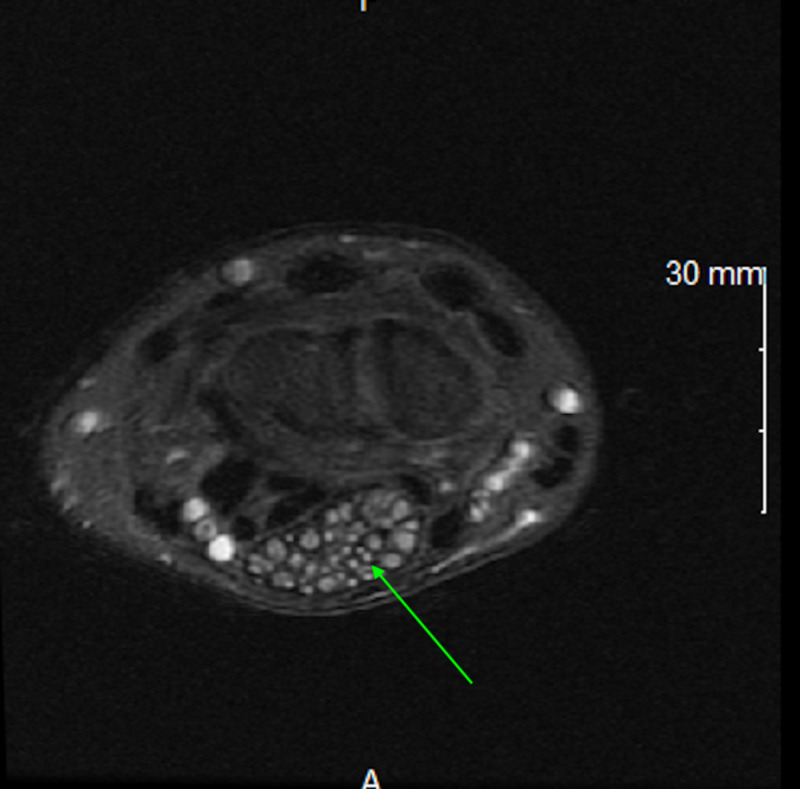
Axial T1 weighted MRI appearance of the right wrist shows 'coaxial cable' appearance in the region of median nerve identified by the green arrow
